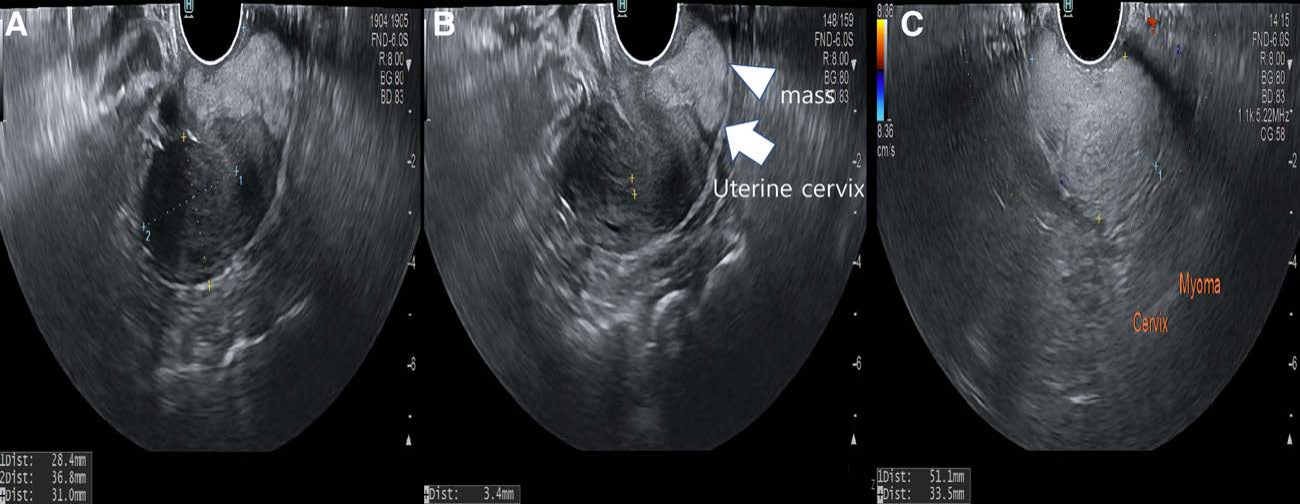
Transvaginal ultrasound findings. (A, B) A well-defined, hyperechogenic mass measuring 58 34 mm ×in size was seen in the cervix. Thickness of the endometrium was 3 mm. Both ovaries and tubes were normal on transvaginal ultrasound. (C) There was no vascularity in the mass on color Doppler.
